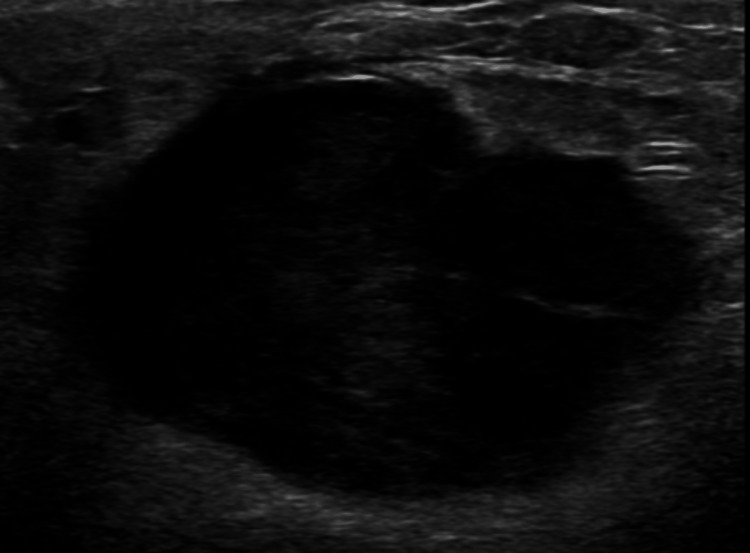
Targeted ultrasound showing a 4 cm irregular, mixed echogenic mass located 7 cm from the nipple corresponding to the palpable mass.
